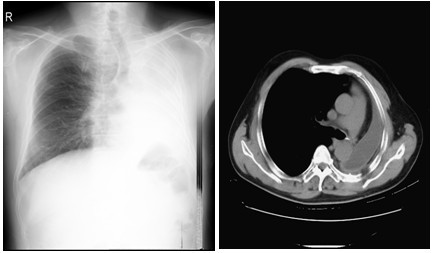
Chest radiograph(Left) and Chest CT (Right) demonstrating the left lung was excised and no lymphatic metastasis.
